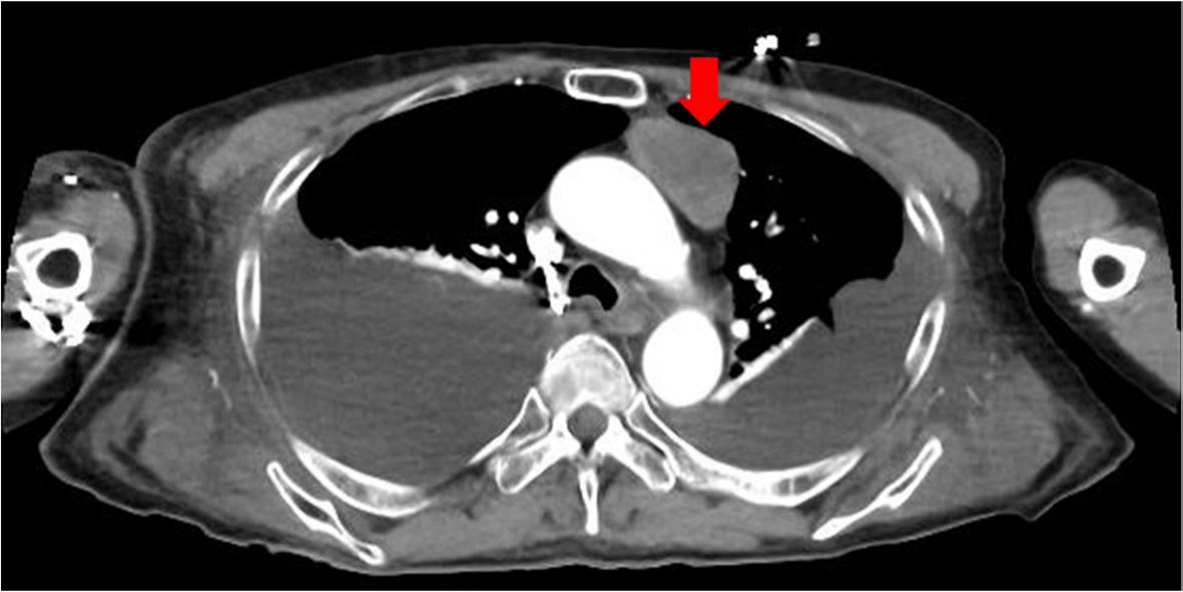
Computed tomography showed a low-attenuation anterior mediastinal mass not invading the surrounding organs
